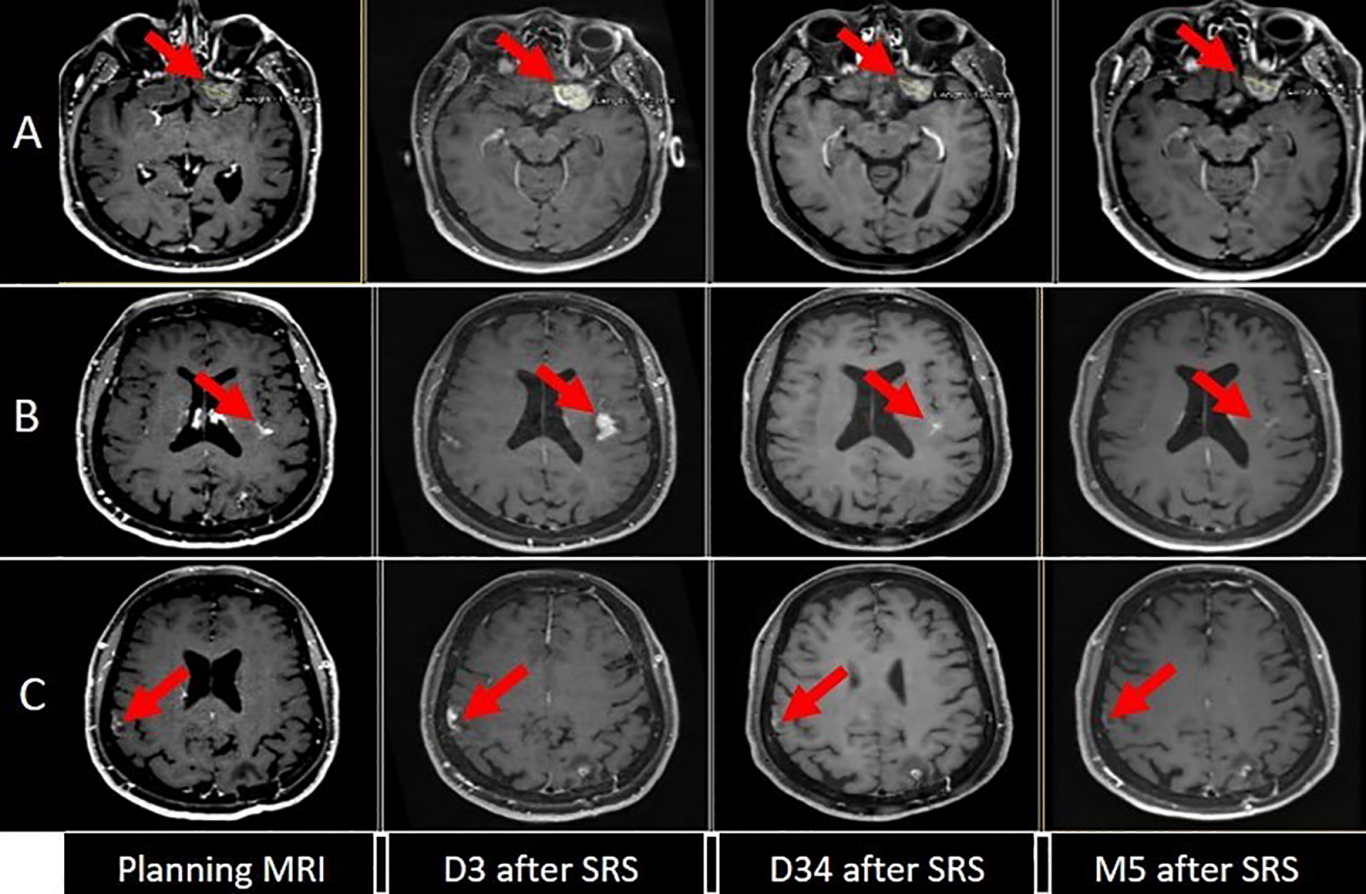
Evolution of brain disease [(A) left fronto-orbital, (B) left posterior insula, (C) right inferior parietal] between planning MRI (one week before SRS), and day 3 (D3), day 34 (D34) and 5 months (M5) after the SRS.
